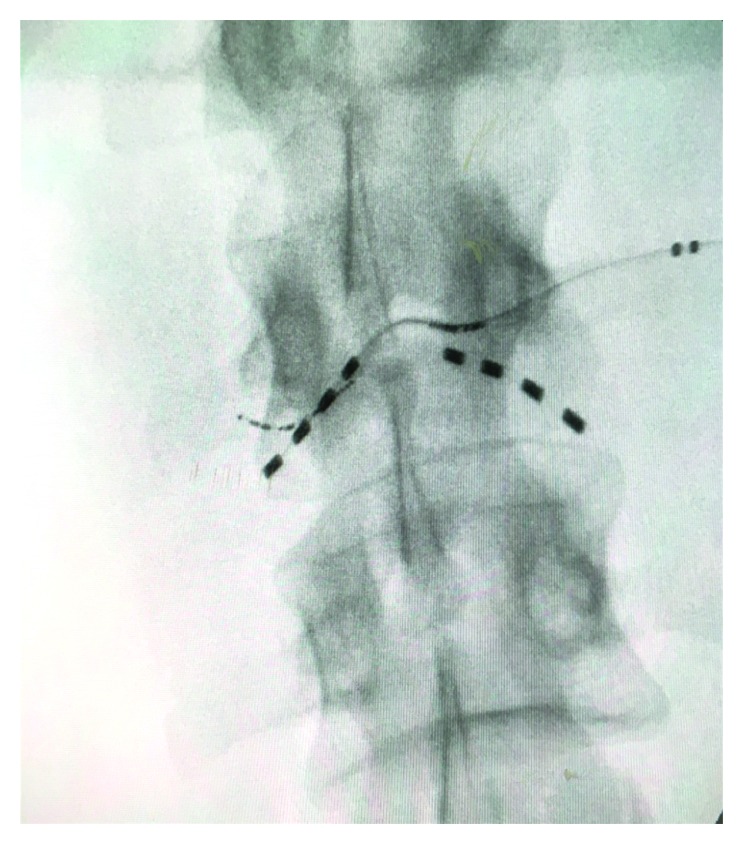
AP view of lumbar spine X-ray showing the electrode placement.
